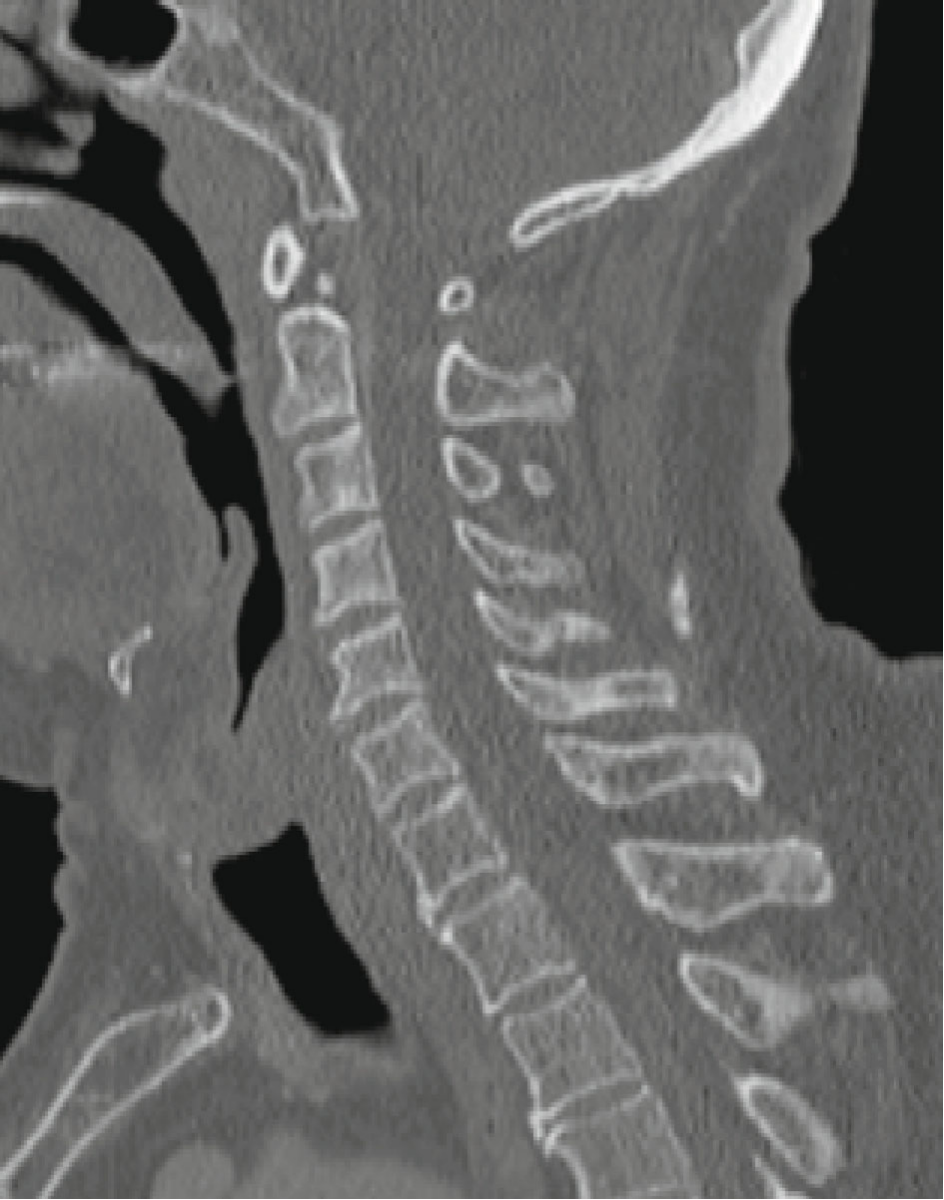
CT of the cervical spine showed no abnormalities.
